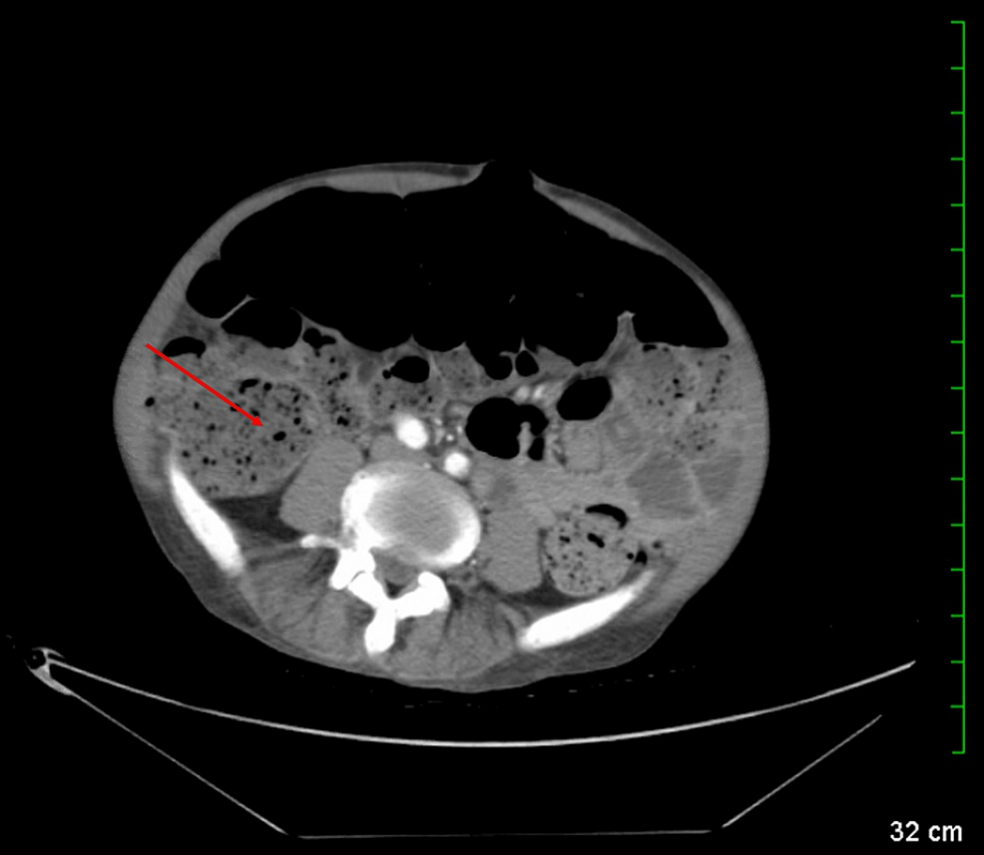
Comparative CT scan of the abdomen and pelvis with IV contrast CT demonstrating impaction with a large amount of stool within a distended colon (red arrow). CT: computed tomography, IV: intravenous
